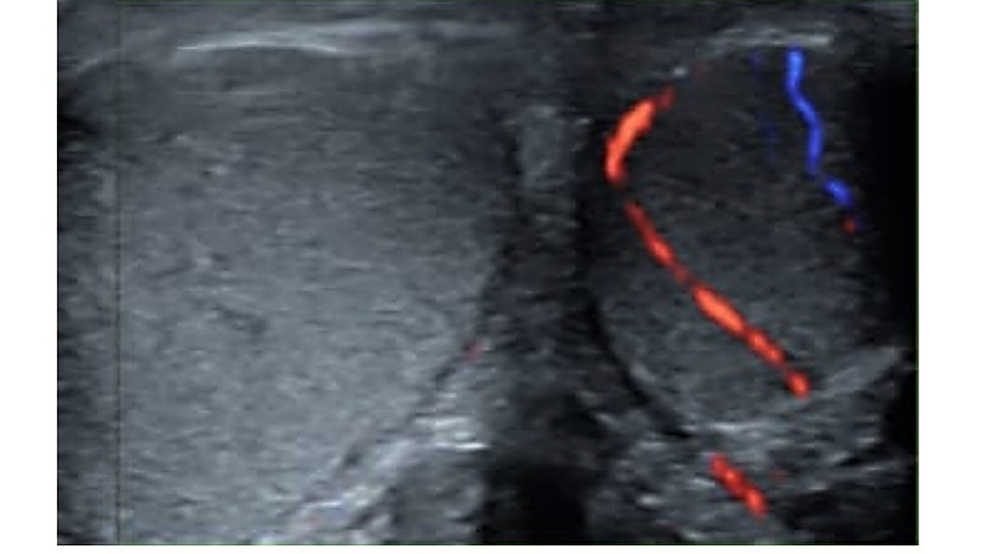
Scrotal Doppler shows no blood flow in the right testis
